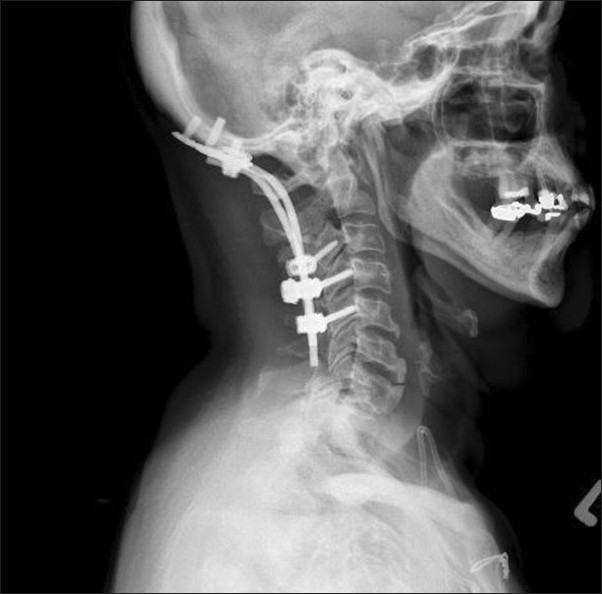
A five-month postoperative lateral X-ray image of the cervical spine shows intact instrumentation without any evidence of hardware loosening, pullout, or failure
